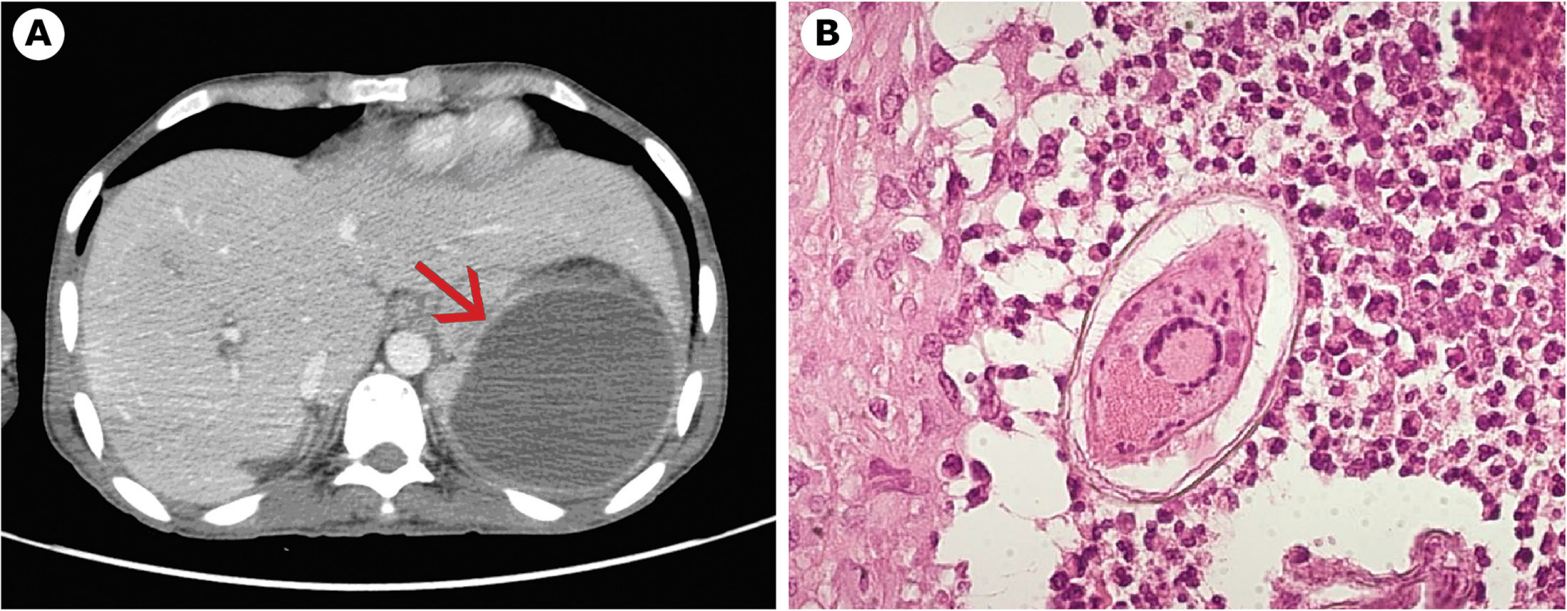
Case 1. a) Splenic lesion at CT scan. b) *Schistosoma mansoni* egg in spleen tissue.
